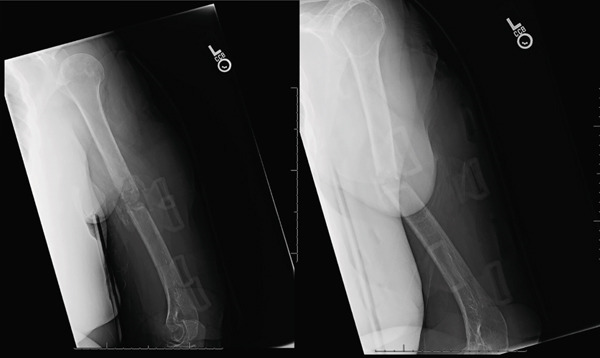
AP and lateral radiographs taken 6 weeks after injury. Radiographs demonstrate interval callus formation with a 23° apex anterior malalignment.
